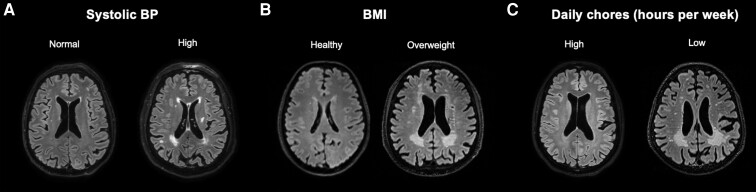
Representative FLAIR axial slices in age- and sex-matched participants stratified by variables significantly associated with WMH load. (A) Systolic BP: 66-year-old male with normal systolic BP of 119 mmHg (left); and 67-year-old male with elevated, clinically hypertensive systolic BP of 152 mmHg (right). (B) BMI: 63-year-old male with BMI of 19.6, falling within the healthy range (18.5–24.9); and 64-year-old male with BMI of 28.5 falling within the overweight range (25–29.9). (C) Sixty-two-year-old male with informant-reported >7 h of chores daily (left); and 63-year-old male with informant-reported no daily chore hours (right). Overall, we found increased WMH load in participants with elevated systolic BP, high BMI and fewer informant-reported daily chore hours.
